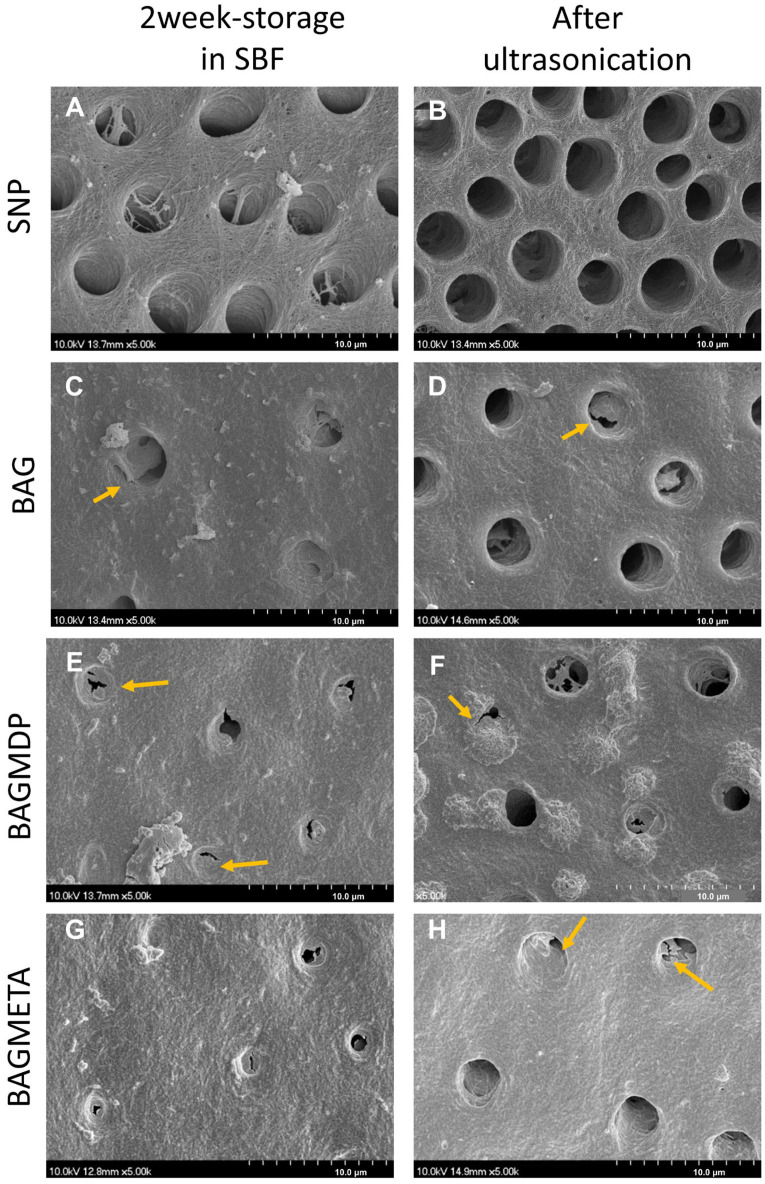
Representative SEM images of dentin surfaces: (A,B) SNP, (C,D) BAG, (E,F) BAGMDP, and (G,H) BAGMETA groups. SNP group (A,B). Left column images show the dentin surface after 2-week storage period in SBF, and right column images show the dentin surface after application of ultrasonication. The images present that the BAG-containing groups occluded exposed dentin surfaces with precipitates (arrows) and those occlusions were retained after the ultrasonication.
